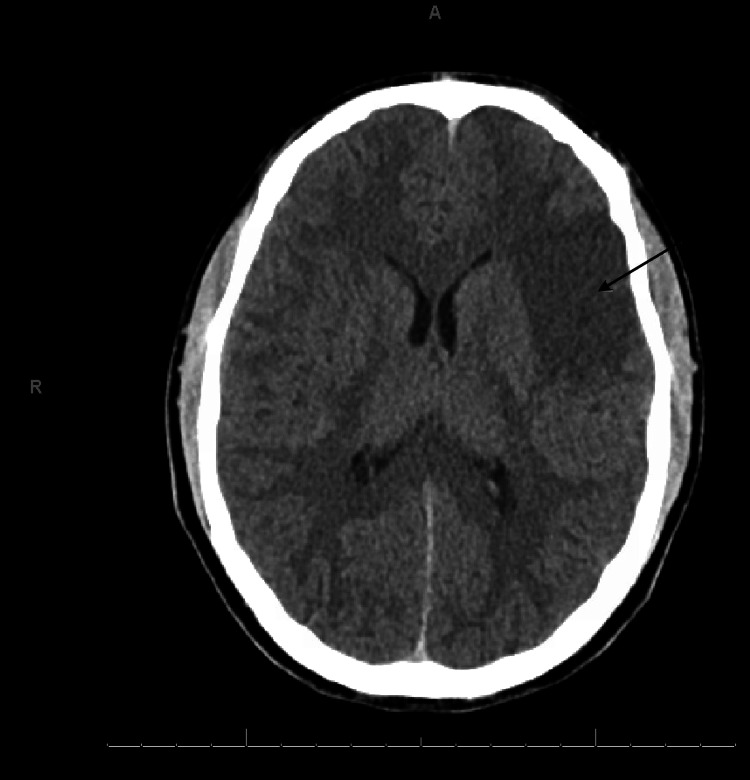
CT brain of the patient Arrow demonstrates left MCA-territory ischemic infarction CT: computed tomography; MCA: middle cerebral artery
